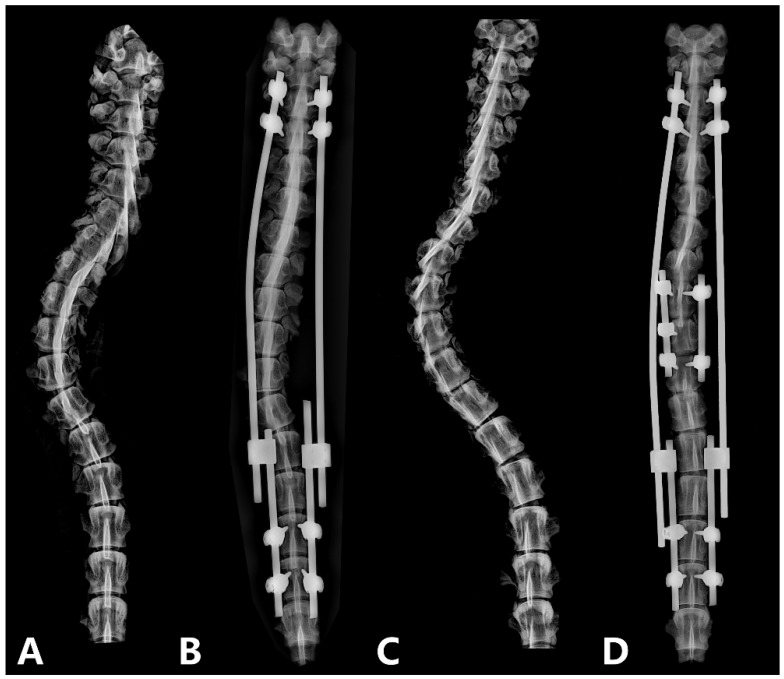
Preoperative and postoperative radiographs of TDGR and HT, (A) preoperative radiographs of TDGR; (B) postoperative radiographs of TDGR; (C) preoperative radiographs of HT; (D) postoperative radiographs of HT.
